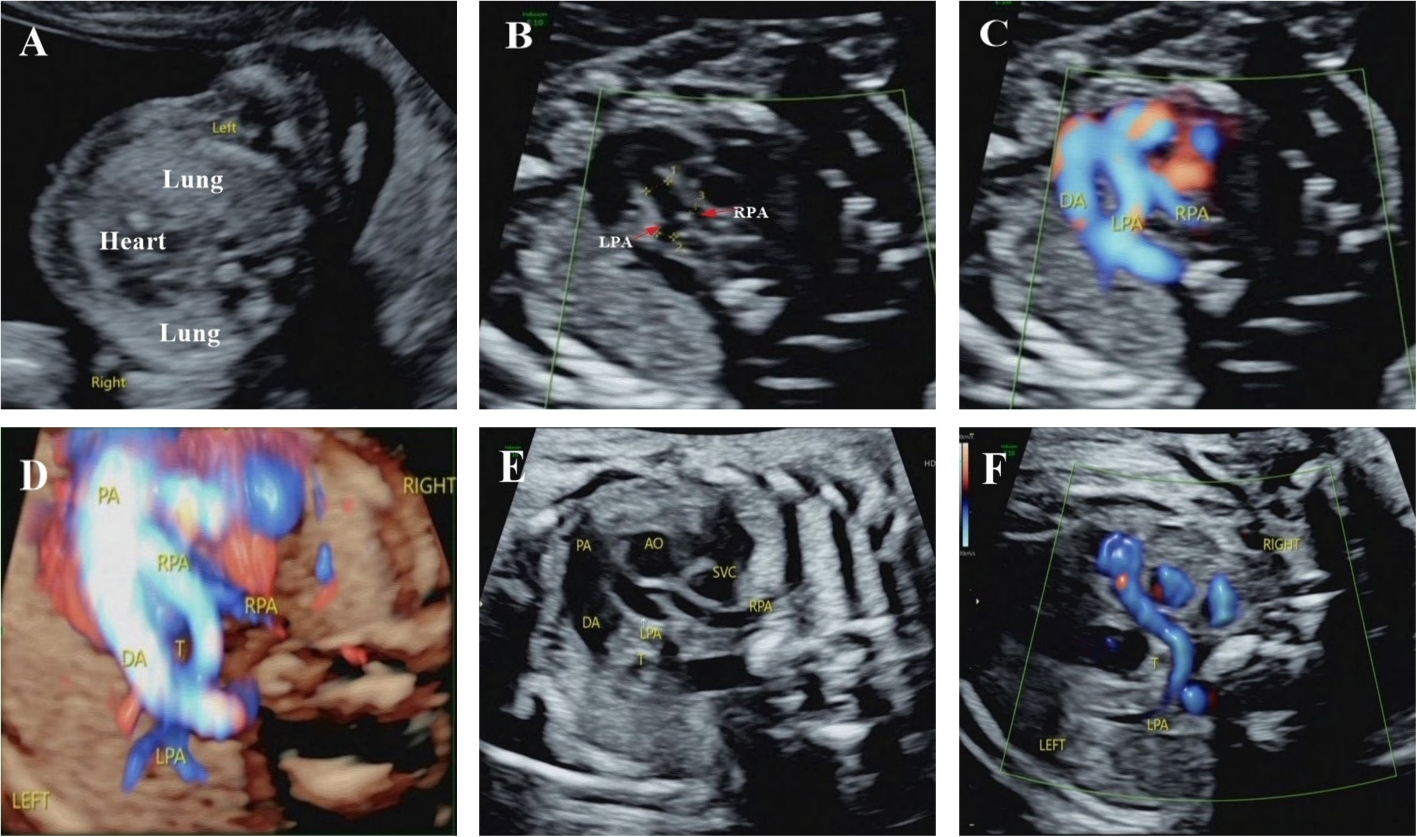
Integrated prenatal and postnatal management of PAS (prenatal portion). (A) Ultrasound examination at 13^+4^ weeks showed that the heart shifted to the right and the volume of the left lung increased compared with the right lung. (B–D) Ultrasound detected PAS at 17^+2^ weeks. Ultrasound examination showed that the left pulmonary artery originated from the right pulmonary artery, bypassed behind the trachea from right to left, and the right pulmonary artery had a narrow inner diameter. These suggested PAS and dysplastic right pulmonary artery. (E,F) Ultrasound examination at 30 weeks confirmed the same diagnosis. PA, pulmonary atresia; DA, ductus arteriosus; AO, aorta; LPA, left pulmonary artery; RPA, right pulmonary artery; SVC, superior vena cava; T, trachea.
